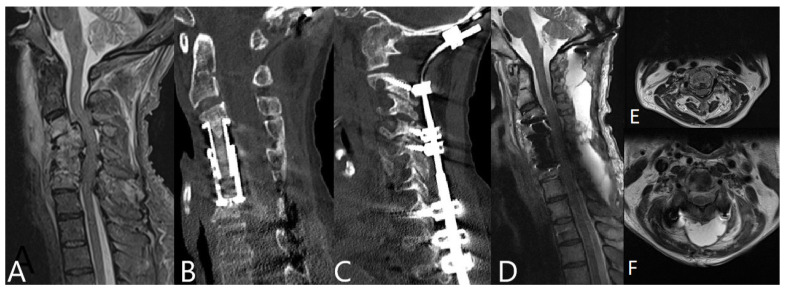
50-year-old male patient with spondylodiscitis due to an infection with methicillin-resistant *Staphylococcus aureus* (MRSA) of C3/4, C4/5, and C5/6, intraspinal and prevertebral empyema, and compression of the spinal cord. Corpectomy of C3, C4, C5, and C6 was performed with implantation of the expandable cage following posterior cervical and thoracic stabilization with lateral mass and pedicle screws with an occiput plate. (A). preoperative T2 weighted MRI of the cervical spine. (B,C). postoperative sagittal CT of the cervical spine shows the spinal construct. (D). postoperative T2 weighted MRI of the cervical spine shows restored alignment without signs of infection 3 months following surgery. (E). T2 weighted MRI of the cervical spine, axial view at C3 level preoperative and (F). postoperative, showing sufficient decompression following ventral decompression and stabilization.
